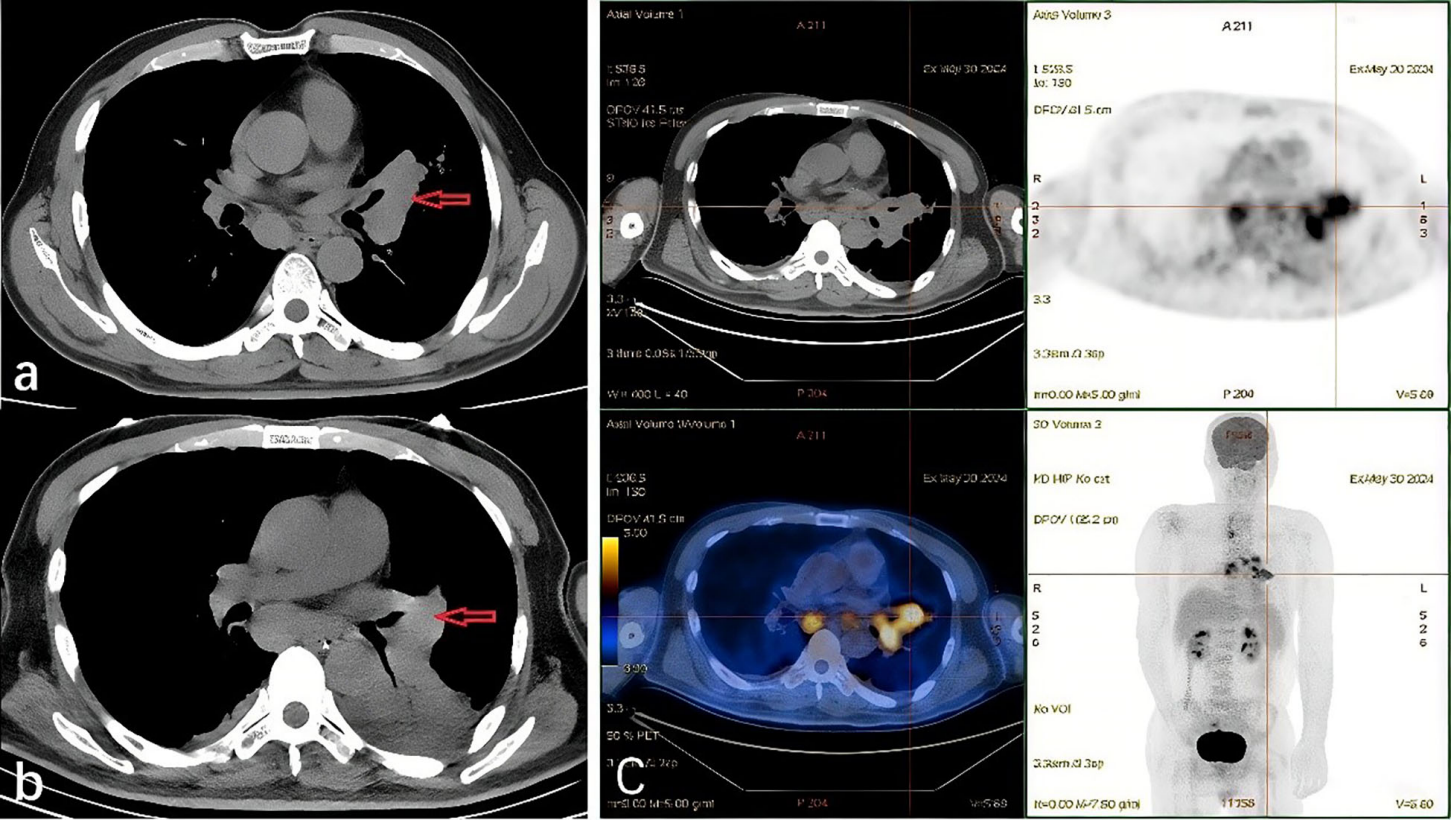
(a) Patient’s chest CT after the admission showed the pulmonary consolidation in the left lung, enlarged lymph nodes in the hilum and mediastinum. (b) Patient’s chest CT after the treatment showed an increased pulmonary mass and lymph nodes. (c) Patient’s Positron Emission Tomography Computed Tomography (PET CT) showed the pulmonary hypermetabolic consolidation (SUVmax=5.69), suggesting malignancy, and there are multiple high metabolic lymph nodes in the mediastinum and pulmonary hilum, indicating metastasis.
